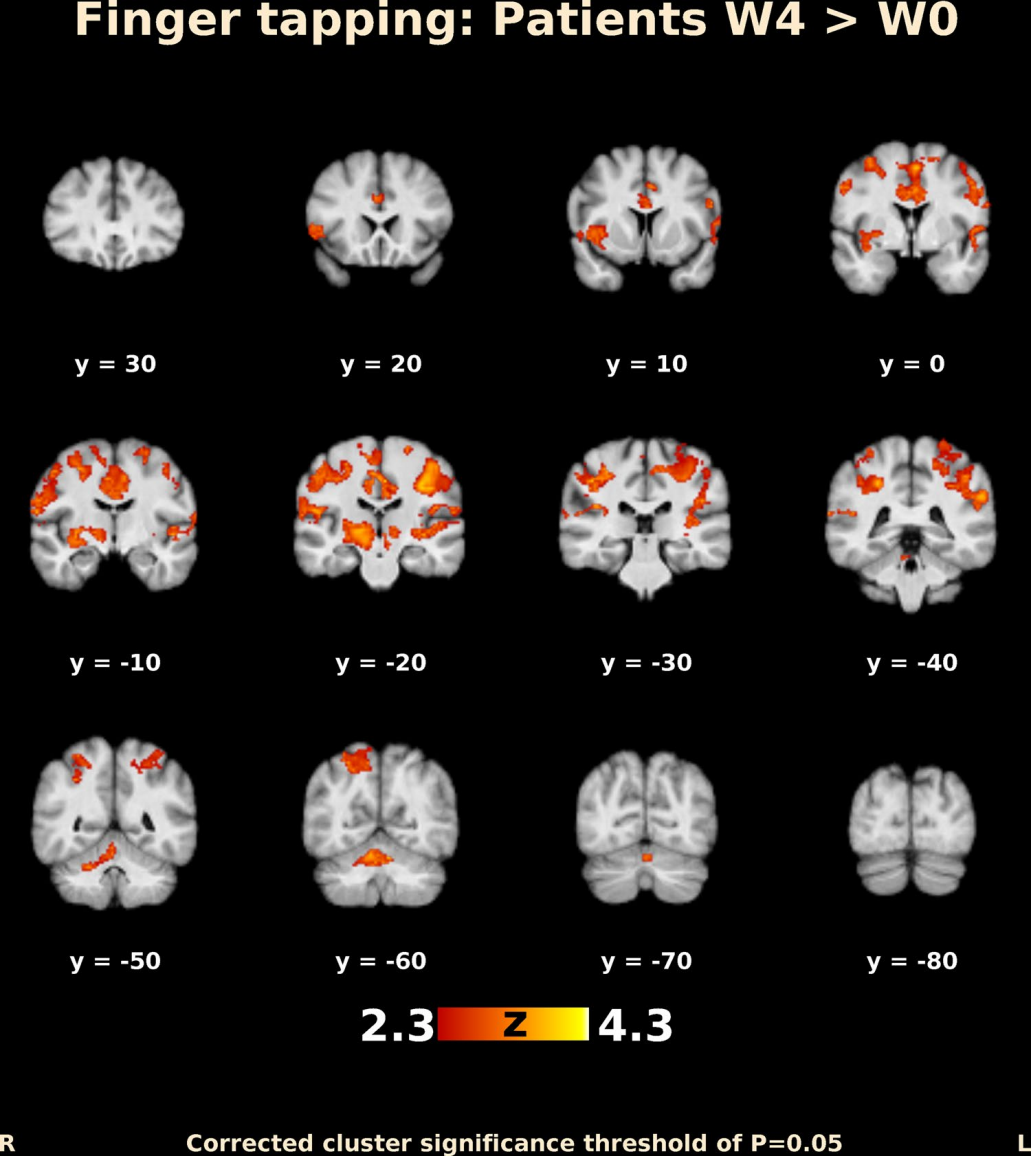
Functional MRI activation map (coronar slices) in patients with CD. Differences in activation after and before BoNT-A injection. Slices are labeled with *Z*/*Y* coordinate in standard MNI152 space
